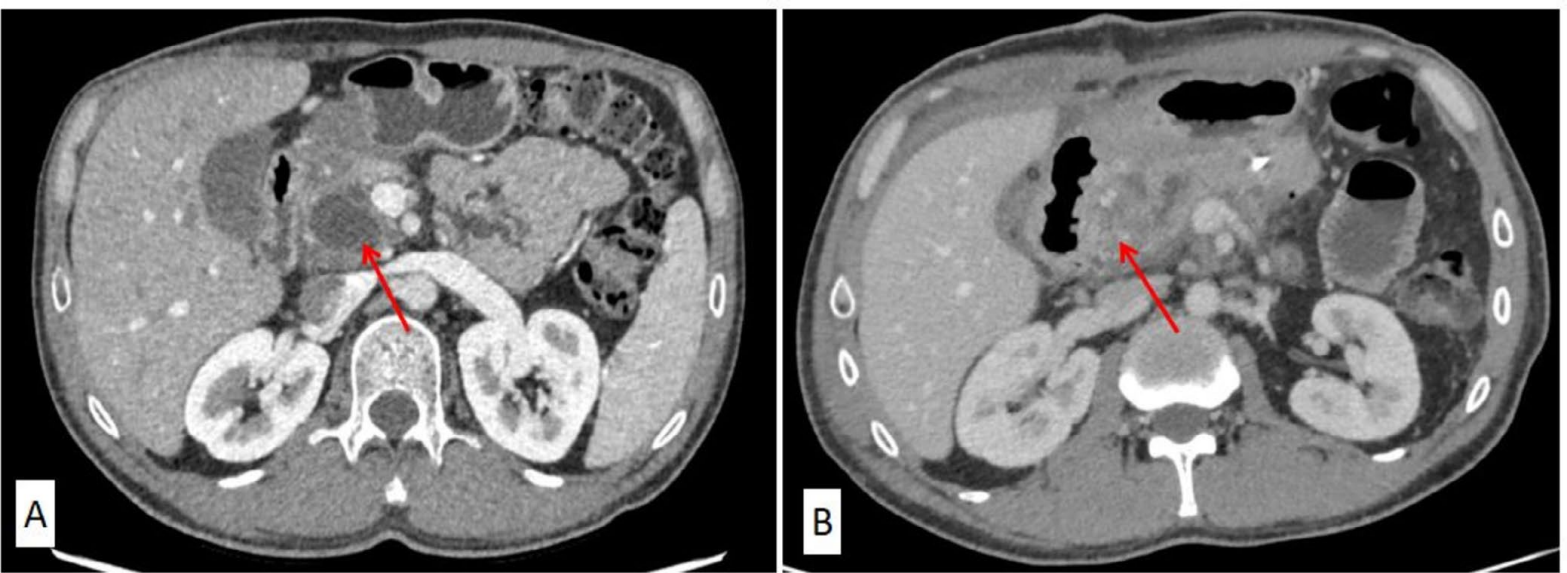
CT scan of pancreatic and intestinal snout area before (A) and after (B) pancreatic cyst surgery.
